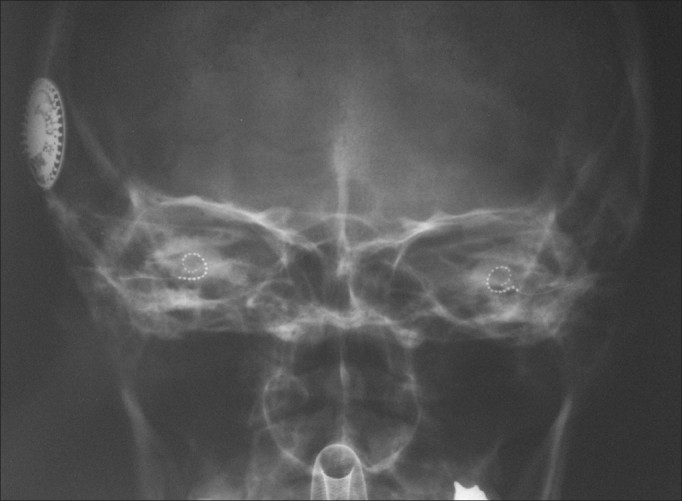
Transorbital x-ray image of the patient at the end of the procedure showing both electrodes inserted in the cochleas.
